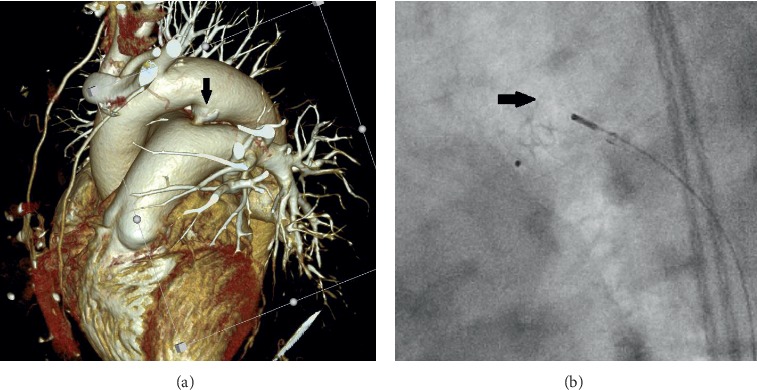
(a) Three-dimensional computed tomography reconstruction of a 5 mm PDA type A with calcifications (arrow). (b) Fluoroscopy in lateral view. 5 × 4 mm Amplatzer duct occluder type II on its delivery cable deployed on the basis of PDA aortic ampulla calcifications (arrow).
